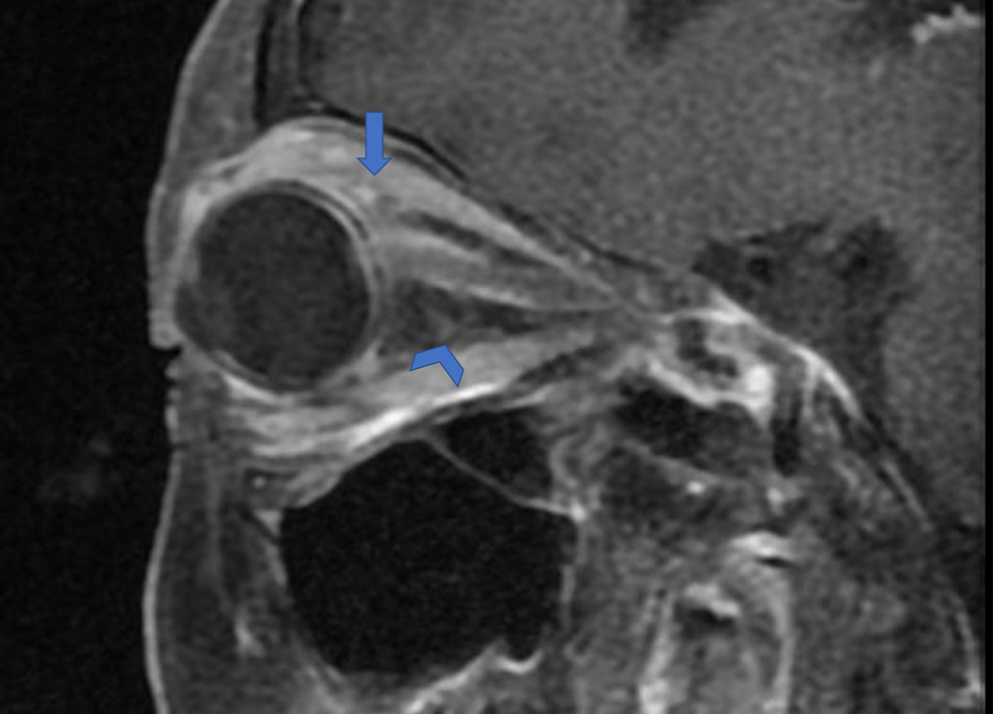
Orbital fat inflammation and inflammatory cell infiltration in OPN. Sagittal contrast enhanced T1-weighted MRI showing orbit fat infiltration (arrowhead) and abnormal contrast enhancement on attachment point of the extraocular muscle (arrow).
